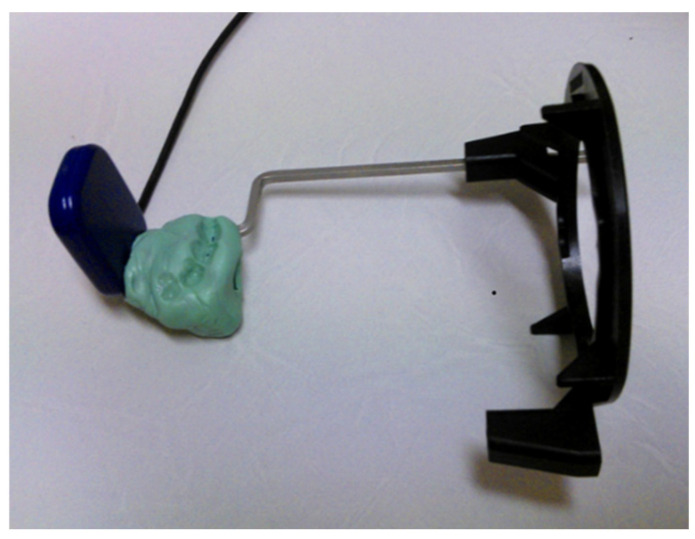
Silicone template for the intraoral X-ray.
